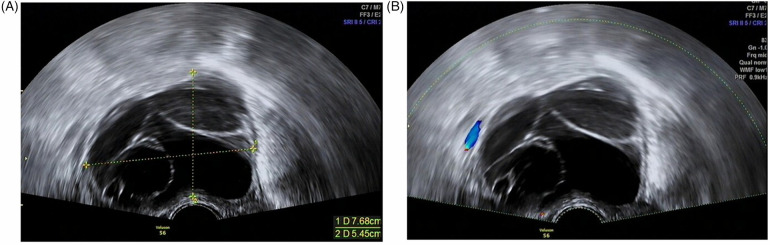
Ultrasound images from a 16-year-old girl with a confirmed mucinous cystadenoma. (A) Conventional ultrasound image shows a 7.7 cm multilocular cyst with smooth inner walls and septations (calibers). (B) Color Doppler image shows no vascularity (Color score = 1). The mass was classified as O-RADS 3.
